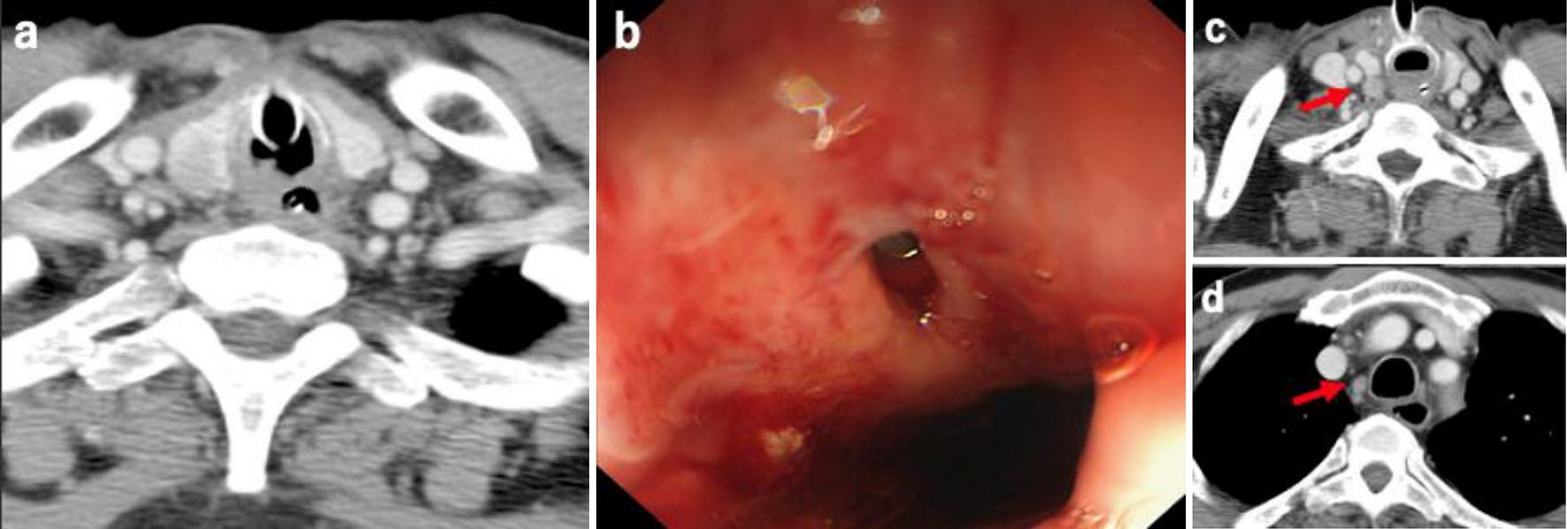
Contrast-enhanced CT and upper endoscopy images after three courses of DGS therapy. The primary tumor achieved complete response on the CT (a) and the TEF remained on the endoscopy (b). All enlarged regional lymph nodes were reduced by more than 30% in their long diameter (red arrows) (c, d) (colour figure online)
